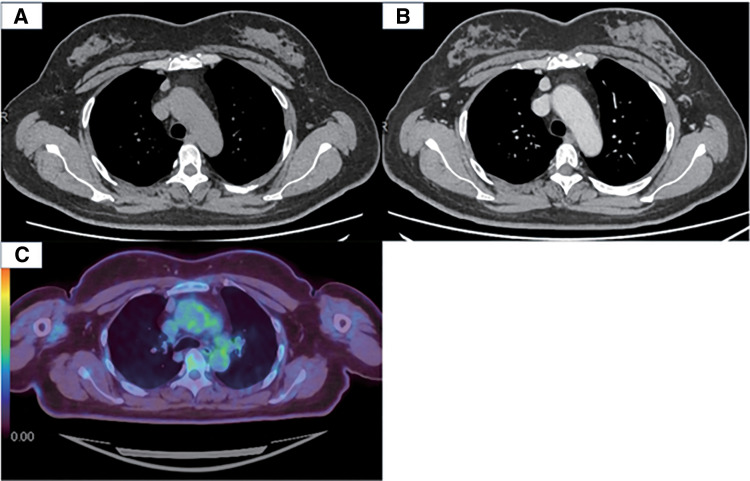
Preoperative chest imaging. (A) CT showed a well-defined, smooth nodule measuring 14 mm on the right side of the anterior mediastinum. (B) Four months later, contrast-enhanced CT showed that the nodule had increased in size to 16 mm. (C) ^18^F-fluorodeoxyglucose-PET/CT showing only a slight ^18^F-fluorodeoxyglucose uptake in the nodular lesion.
